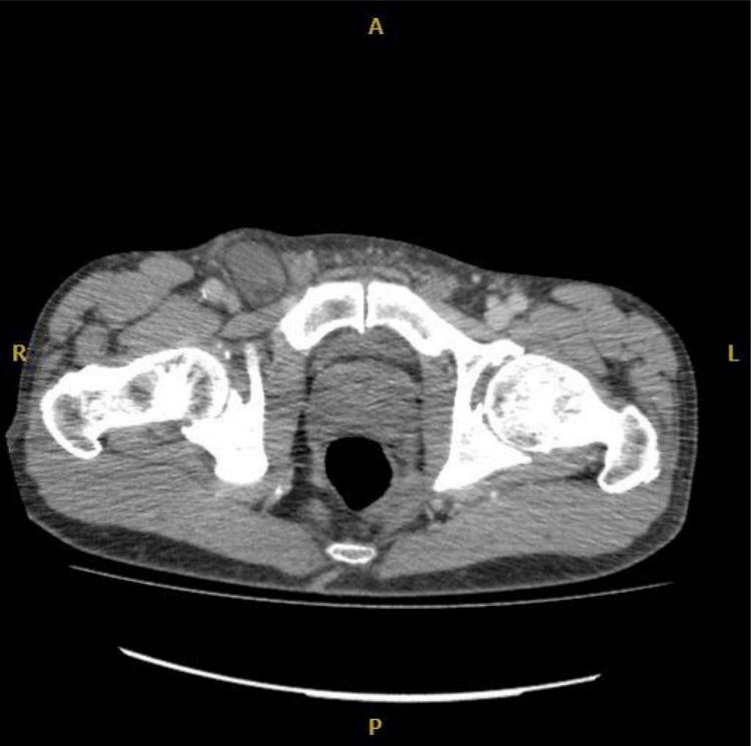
Axial CT image of right De Garengeot hernia.
